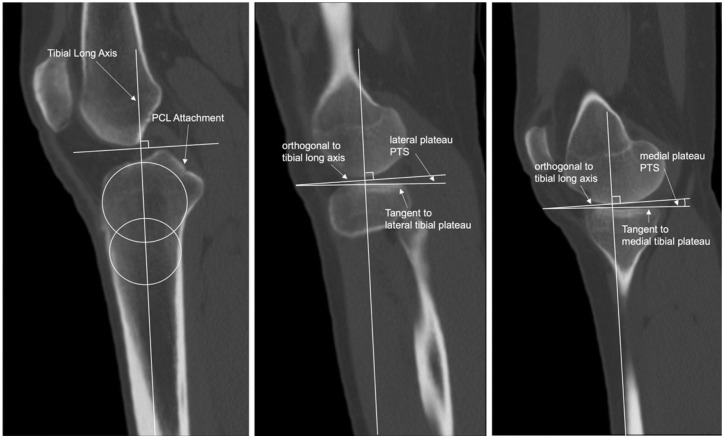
Representative images demonstrating the measurement of the posterior tibial slope (PTS) on computed tomography images in a left knee presented from left to right. Following the method by Hudek etal,^
